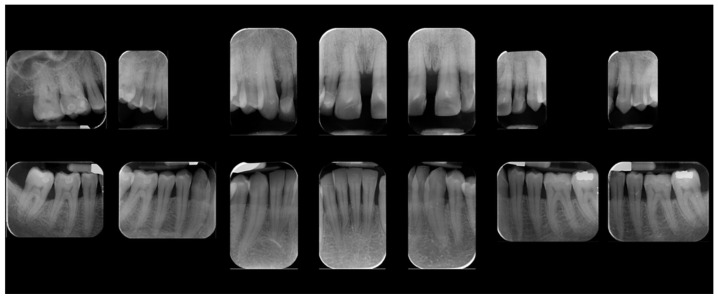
Initial radiographic assessment.
